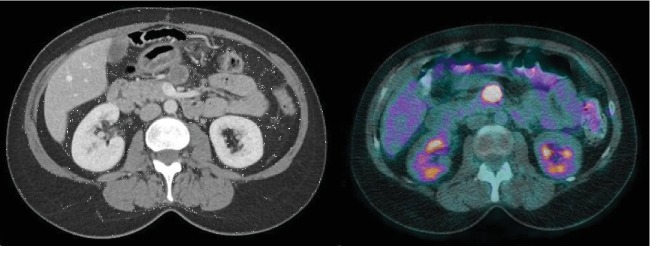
Follow-up abdominoplevic CT scan revealing a suspicious enlarged 2 cm mesenteric lymph node and PET-scan showing a high FDG uptake of the enlarged mesenteric lymph node.
